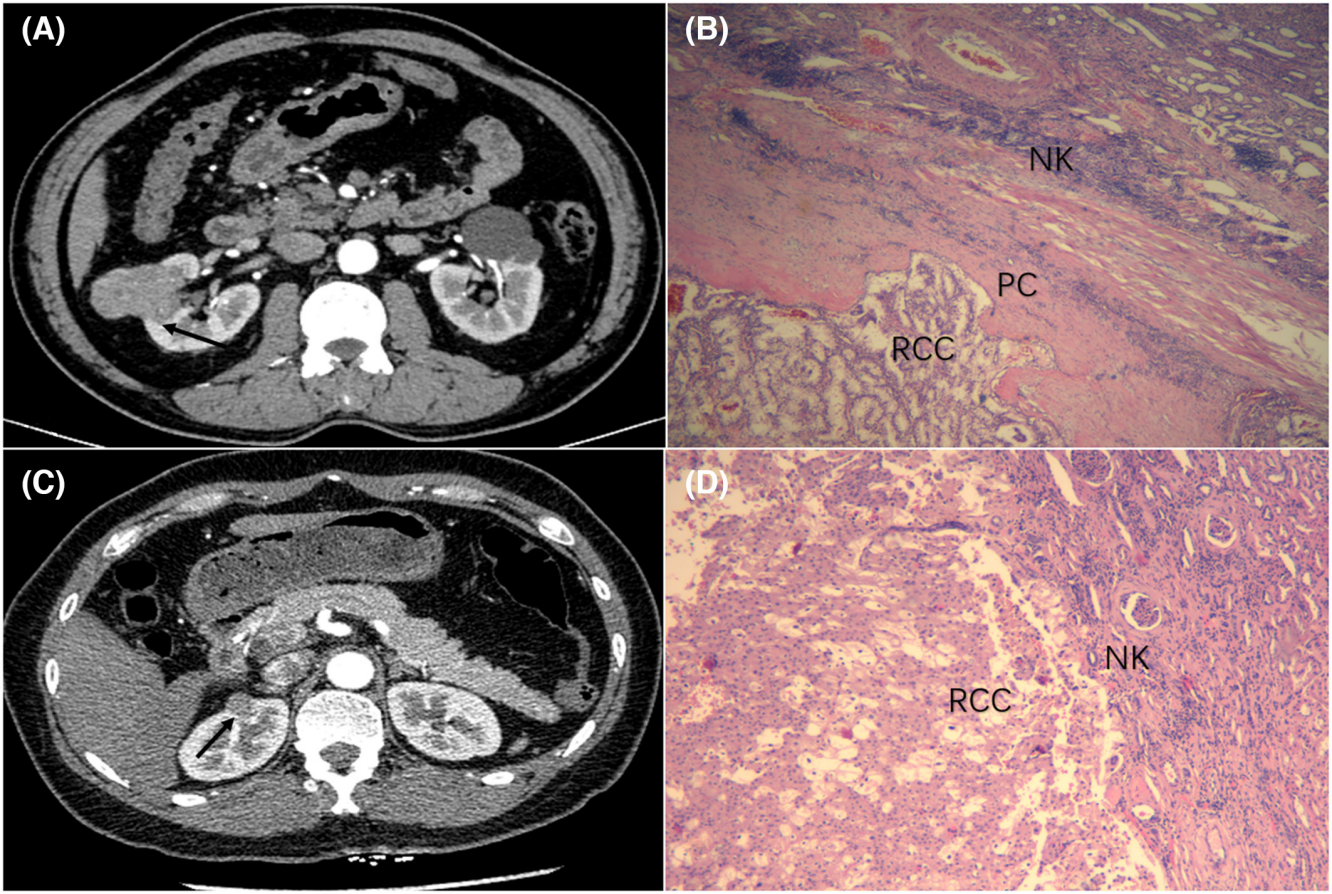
The typical computed tomography imaging feature and PC status in the papillary renal cell carcinoma and chromophobe renal cell carcinoma, respectively (A–D). The irregular tumor shows the hypoenhancement pattern and unclear boundary (arrows) on the corticomedullary phases image (A). Infiltrative tumor cells invade the surrounding pseudocapsule (B). The small tumor shows the hypoenhancement pattern and unclear boundary (arrows) on the corticomedullary phases image (C). The tumor pseudocapsule is completely disappeared at the tumor‐parenchyma interface. NK, normal kidney; RCC, renal cell carcinoma; PC, pseudocapsule
